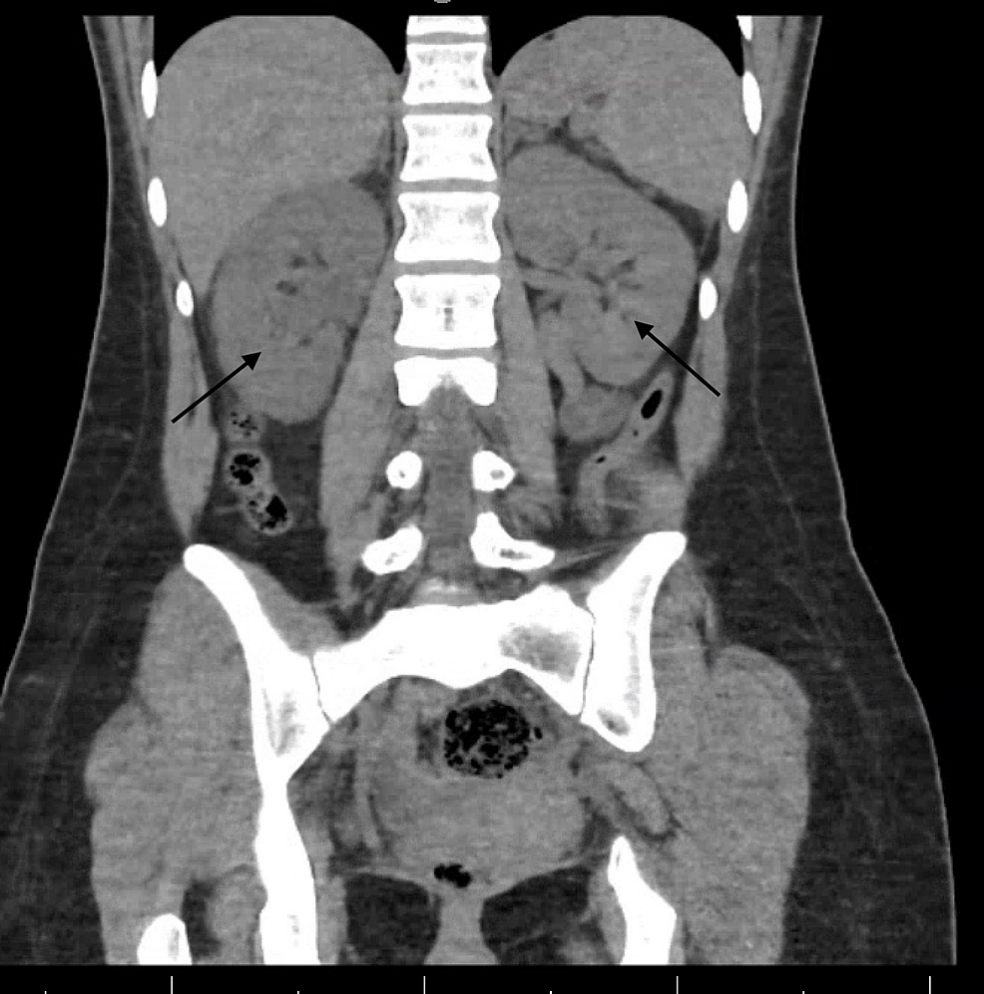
Coronal view of CT abdomen pelvis without contrast showing mild right hydronephrosis. Note the pelvicalyceal dilatation of the right kidney, whereas the left kidney is completely decompressed
